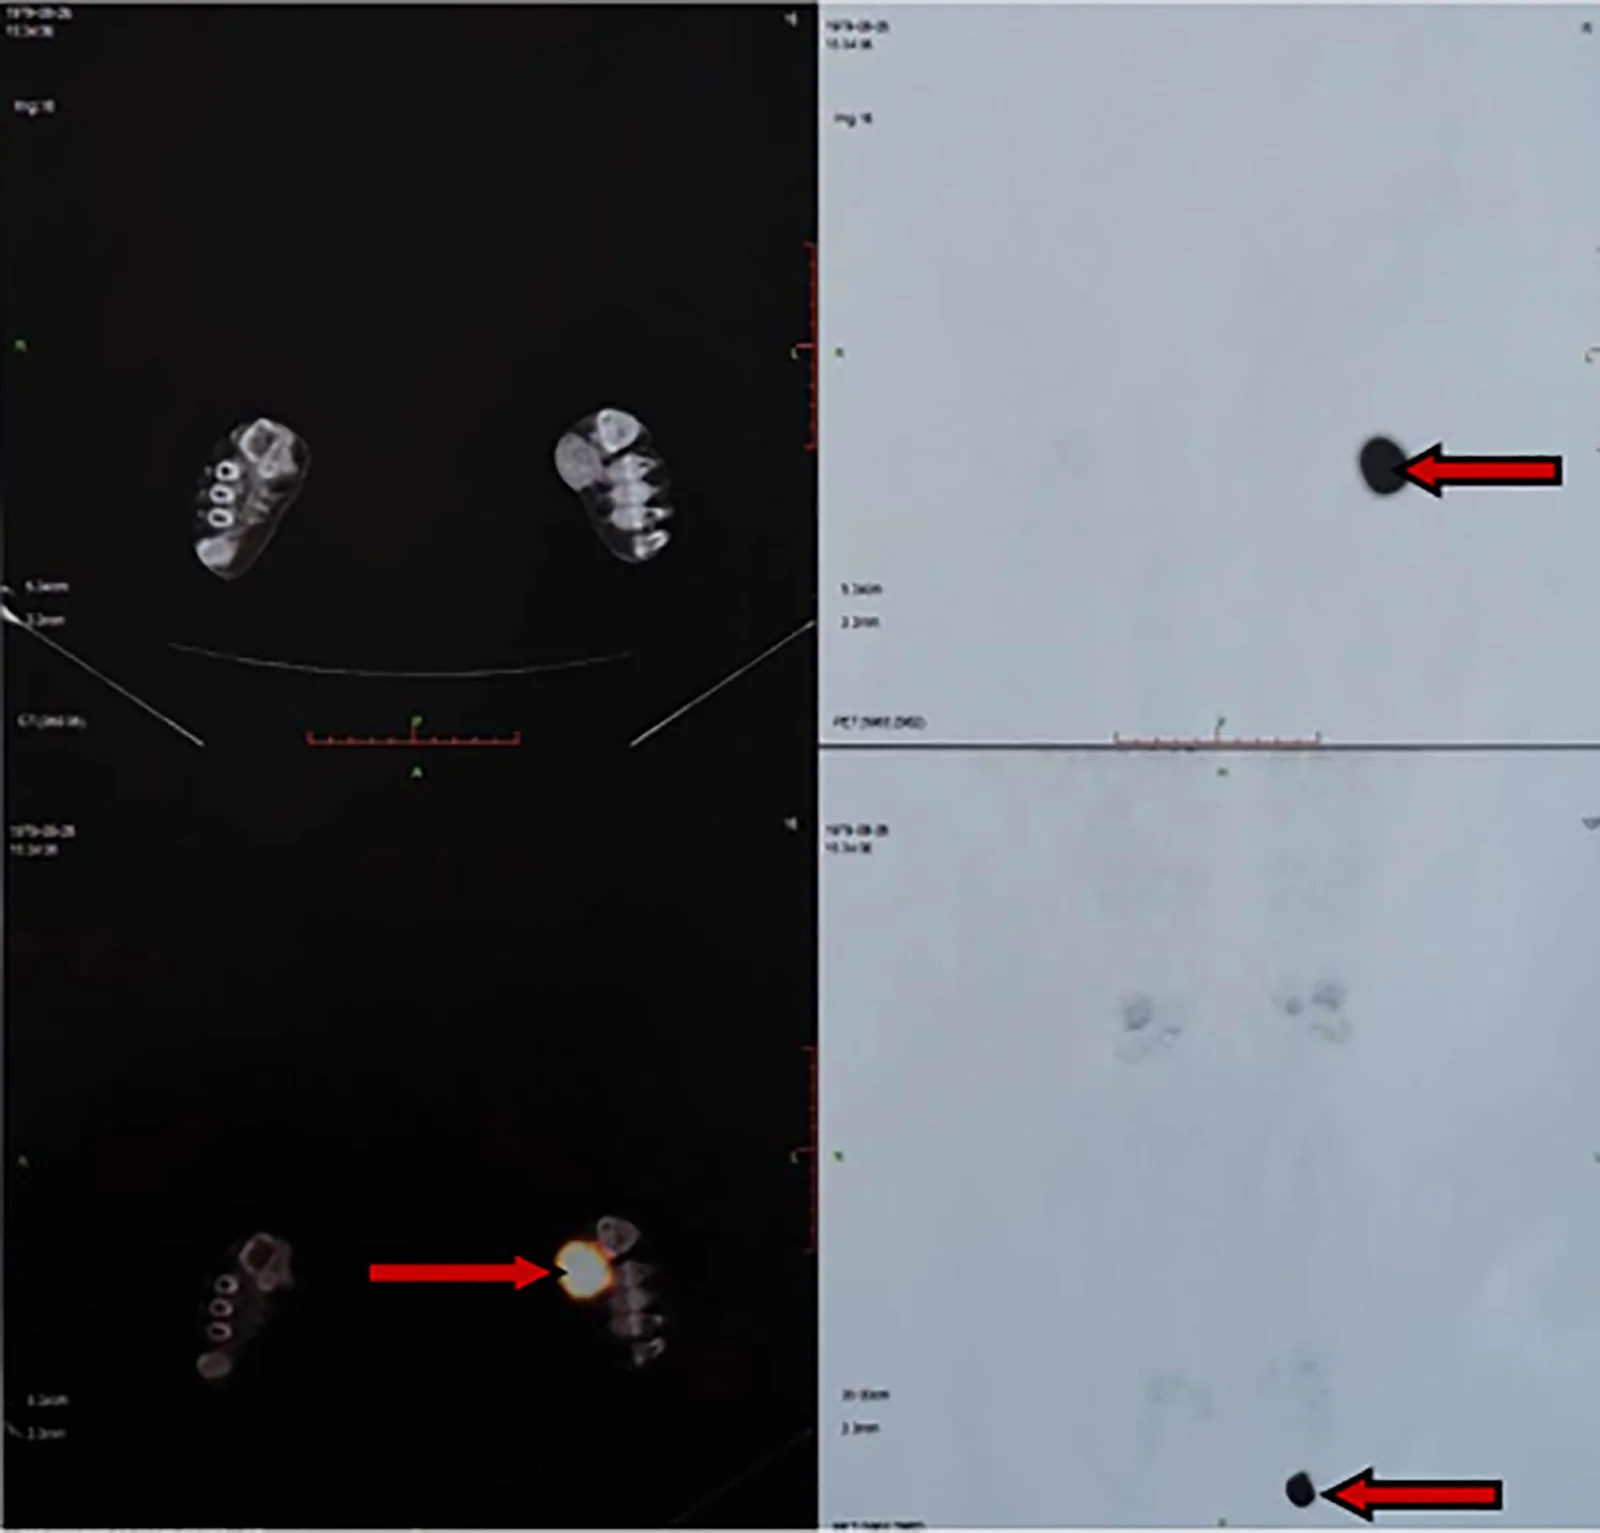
^68^Ga-DOTATATE PET/CT revealed an irregular soft tissue nodule deep in the left plantar first metatarsal, which demonstrated intense DOTATATE uptake.
